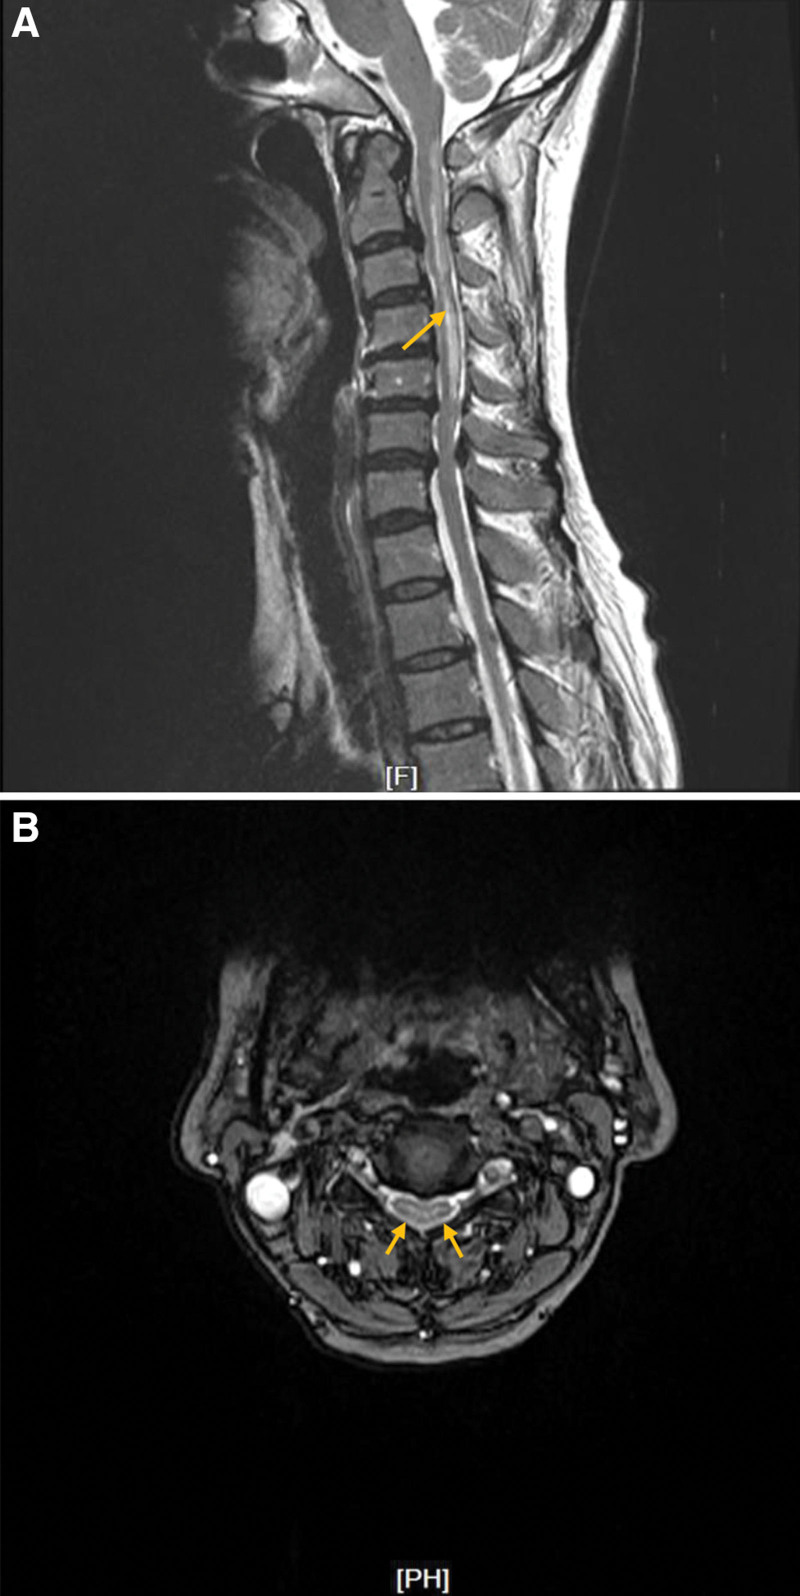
The cervical spine magnetic resonance imaging. A: A T2-weighted image showing the high-intensity area in the posterior column at C2 through C5 (yellow arrow). B: An axial T2-weighted image of the cervical spinal cord showing bilaterally symmetrical high-intensity area within the posterior column (yellow arrows).
